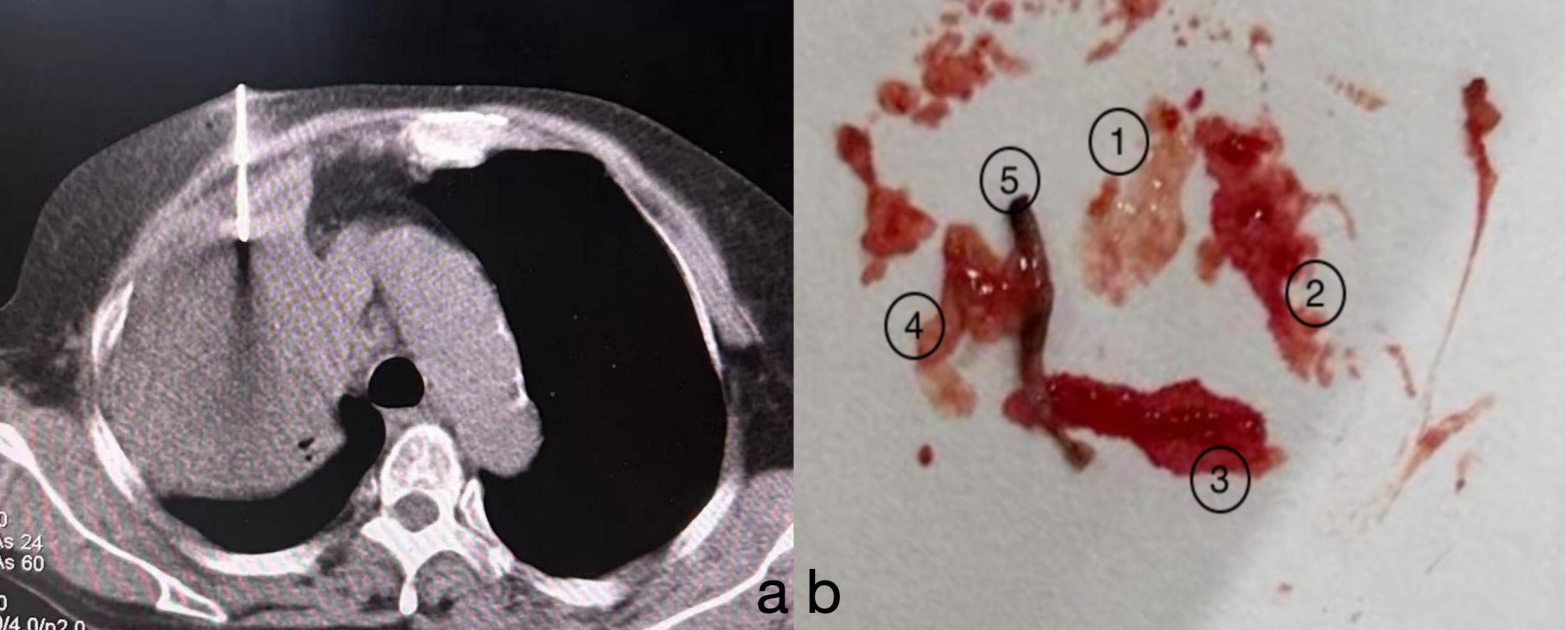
(A) After confirming the puncture site, a biopsy was performed on the lesion under CT guidance from central, anterior, posterior, left, and right angles. (B) A total of five biopsy samples were obtained. Samples 1 to 4 appeared as “fish-flesh-like” tissue, suggestive of necrosis. The fifth sample appeared greyish-white and was considered to be solid tissue from the lesion.
